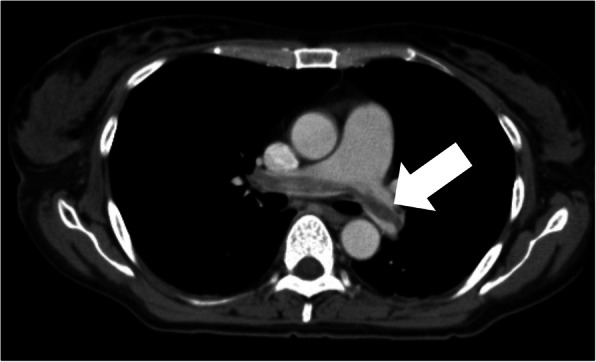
Chest contrast-enhanced computed tomography shows a giant thrombus in the pulmonary artery (arrow)
